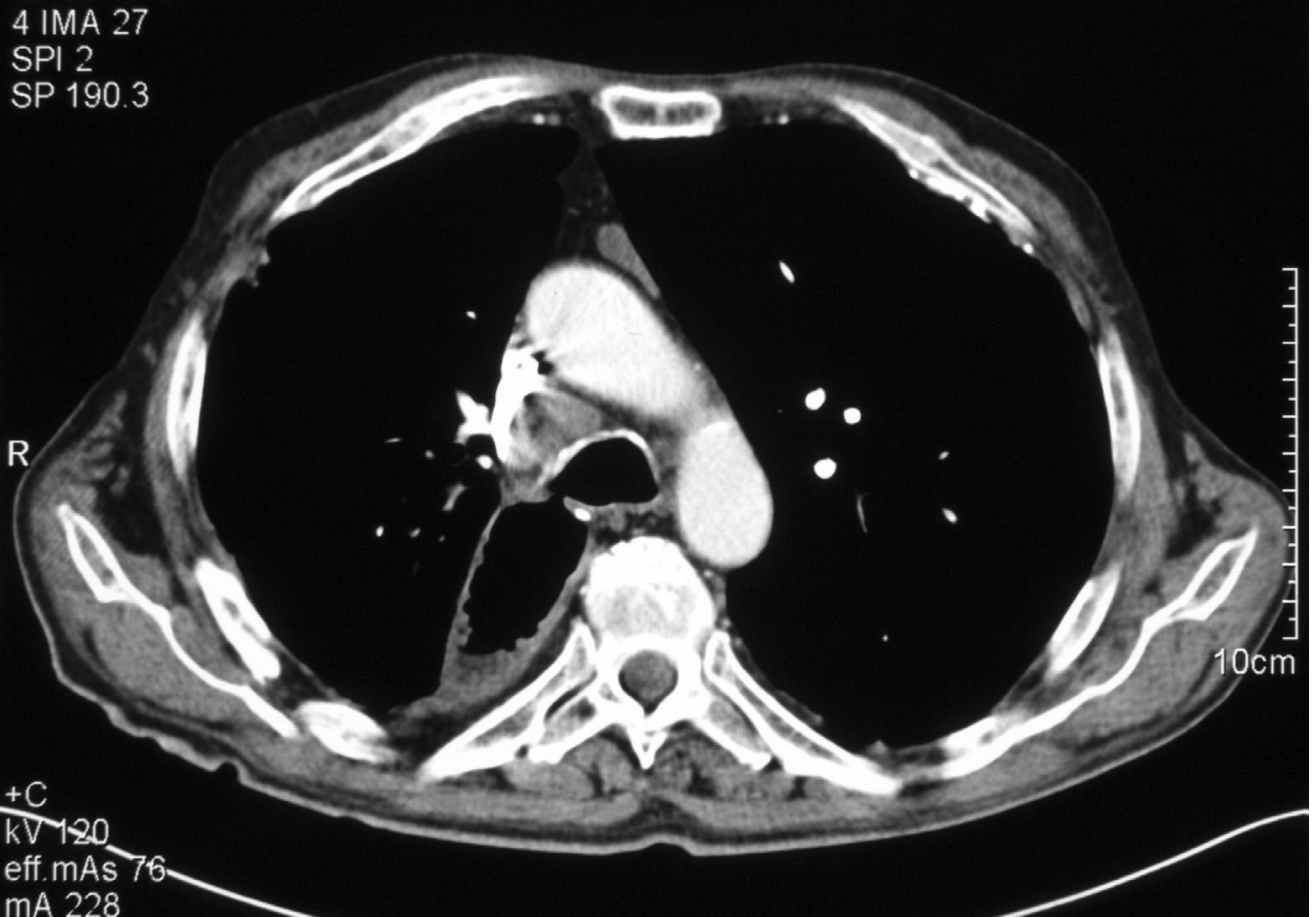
CT scan of chest of showing communication between the right main bronchus and the gastric conduit.
